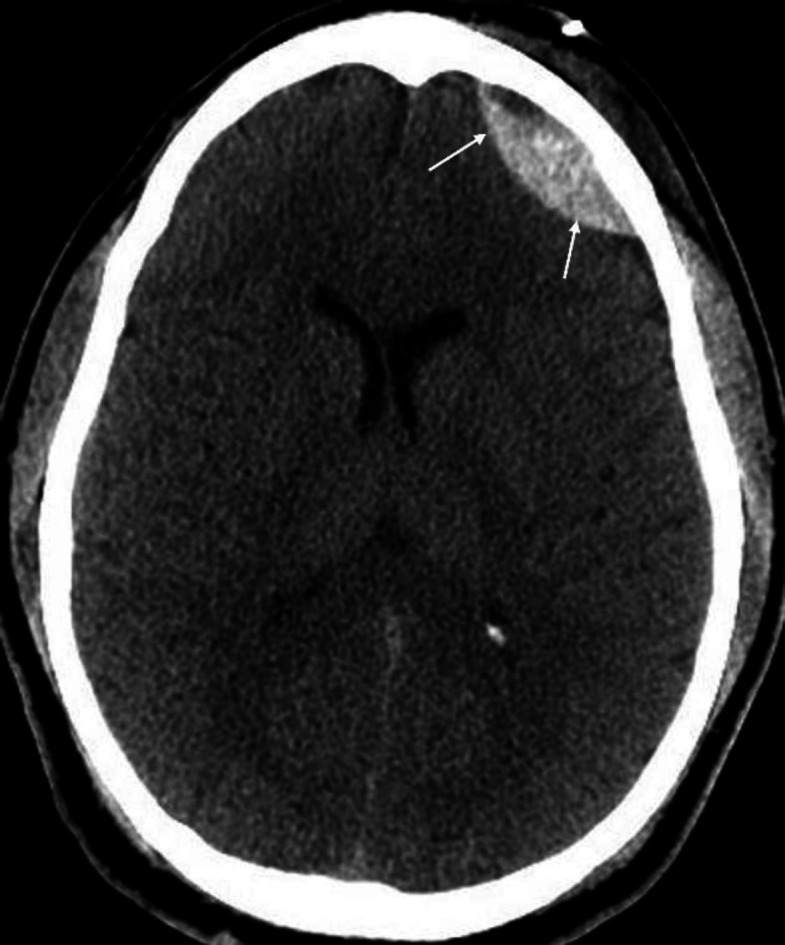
Non-contrast axial CT brain of a 31 years-old male with an epidural (extra-dural) hematoma (arrows) shows a bi-convex hyperdense area in the left frontal region. Typically, an epidural hematoma is lentiform (bi-convex, lens shaped, lemon shaped etc.) and does not cross the sutures. In comparison, a subdural hematoma is cresenteric (moon shaped, sickle shaped, banana shaped etc.) and can cross the suture or midline
